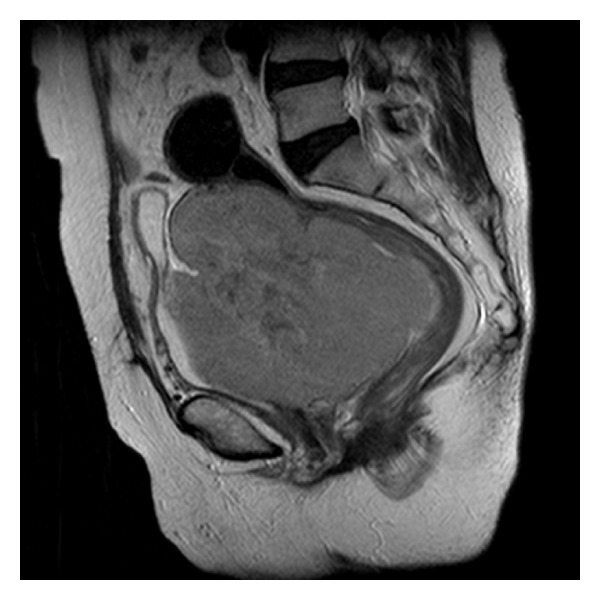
Sagittal T2-weighted magnetic resonance imaging showing a large pelvic mass (13 × 13 × 11 cm) compressing the posterior bladder wall.
